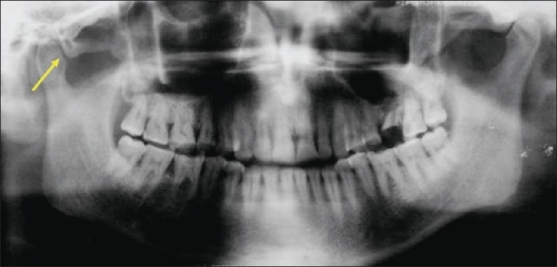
Panoramic radiograph shows a unilateral (R) multilocular ZACD (arrow)
